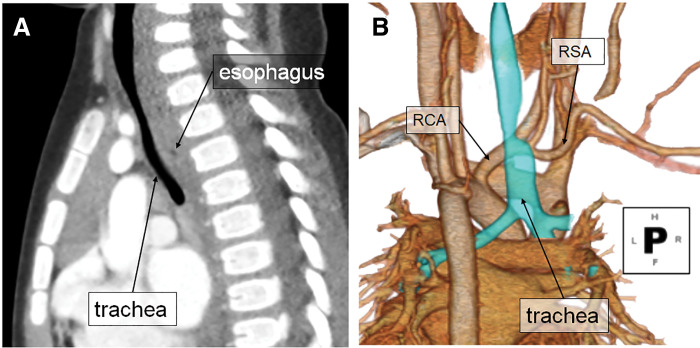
Images of CT scan examined 6 months after surgical intervention. ARSA was not detected. The RSA is anastomosed to the RCA and passes anterior to the trachea. The esophageal dilation had been reduced, and tracheal stenosis had been reduced. (A) Sagittal view (B) 3D reconstruction image. ARSA, aberrant right subclavian artery; RCA, right common carotid artery; RSA, right subclavian artery
